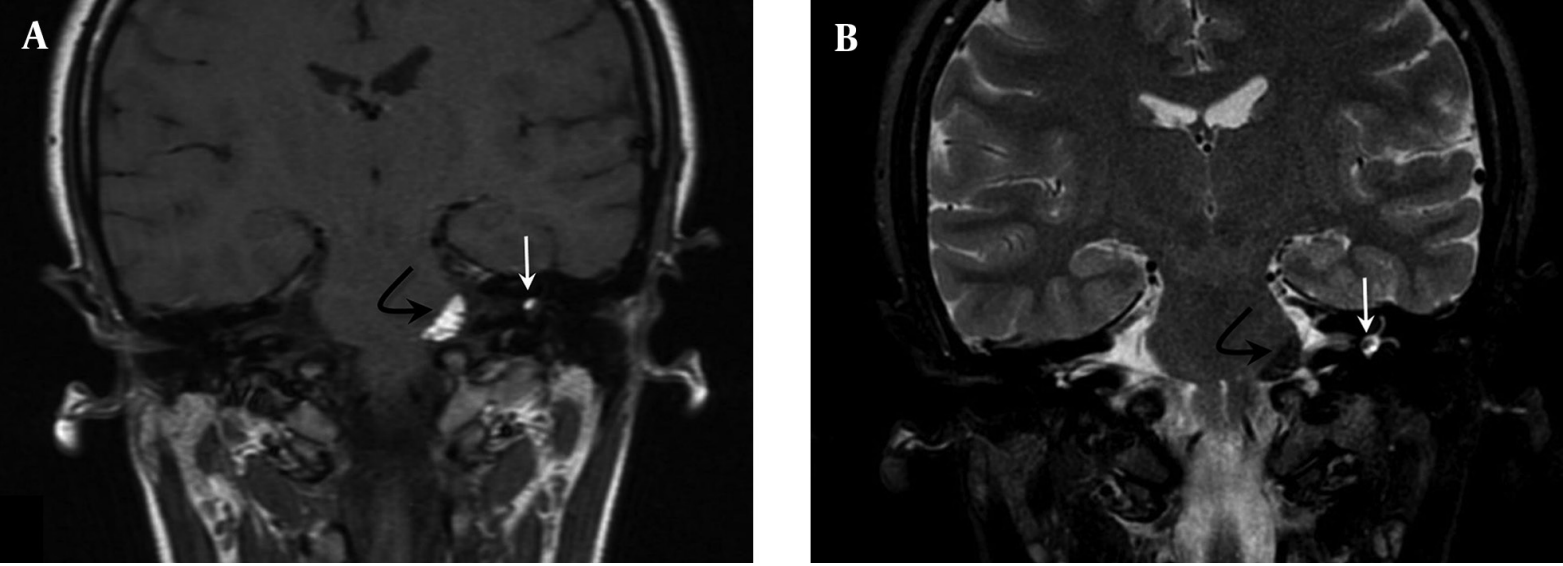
A) Coronal T1-weighted MRI image reveals hyperintense lesions in the left cerebellopontine angle (black arrow) and the left internal acoustic canal (white arrow); B) Coronal T2-weighted fat suppressed image reveals saturated hypointense lesions.
